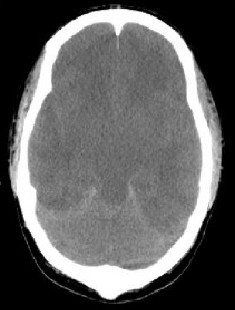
Computerized Tomography (CT) head showing diffuse cerebral edema with effacement of basal cisterns and generalized loss of gray-white differentiation
